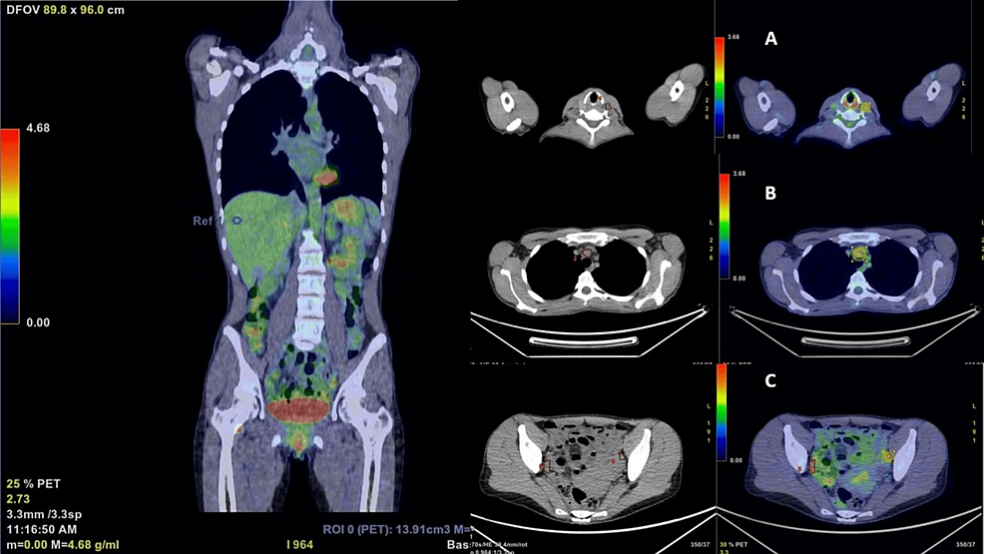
Abnormal concentration of the FDG A: carotid arteries (SUV=3.2); B: aortic arch (SUV=3.2); C: Iliac arteries (SUV=5.6) FDG - fluorodeoxyglucose; SUV - standardized uptake value
